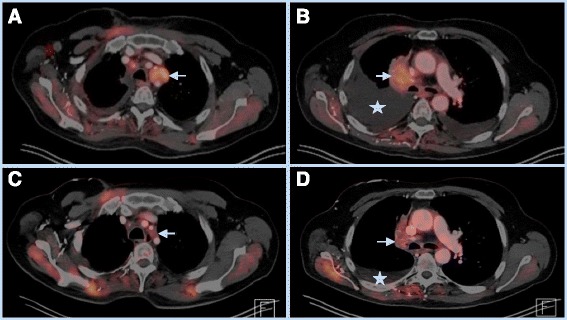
Top*- PET-CT images July 2015 showing 3.2 cm left para-tracheal mass with SUV 6.8 (a) and 4.6 × 3.1 cm right para-tracheal mass with SUV 6.5 (b). Also seen are large right and small left pleural effusions. *Bottom*- PET-CT images from December 2015 showing complete resolution of left para-tracheal mass, and decreased size of right para-tracheal mass with equivocal hypermetabolism (SUV 2.6) along with complete resolution of left pleural effusion and residual small right pleural effusion
